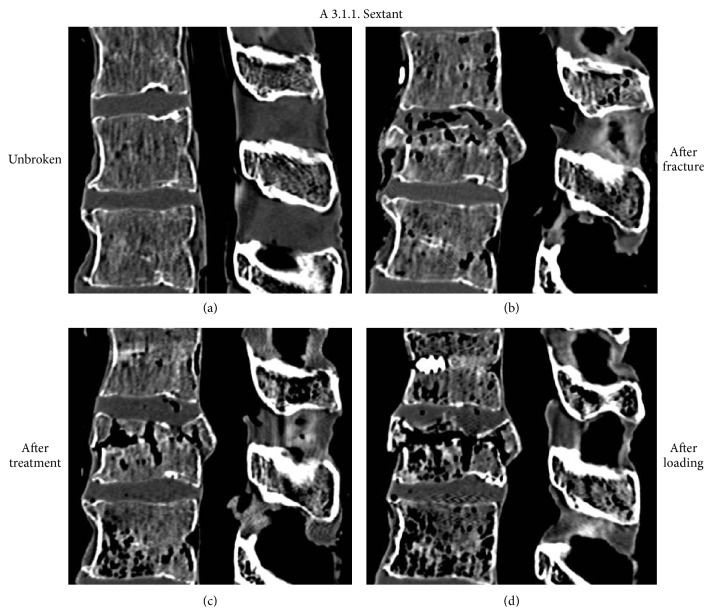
Representative case of an incomplete cranial burst fracture A 3.1.1. treated with sole dorsal instrumentation. CT scans in the midsagittal plane before (a) and after (b) fracture, as well as after treatment (c) and after cyclic loading (d). Note that after treatment limited height restoration was achieved. This could not be maintained after cyclic loading.
